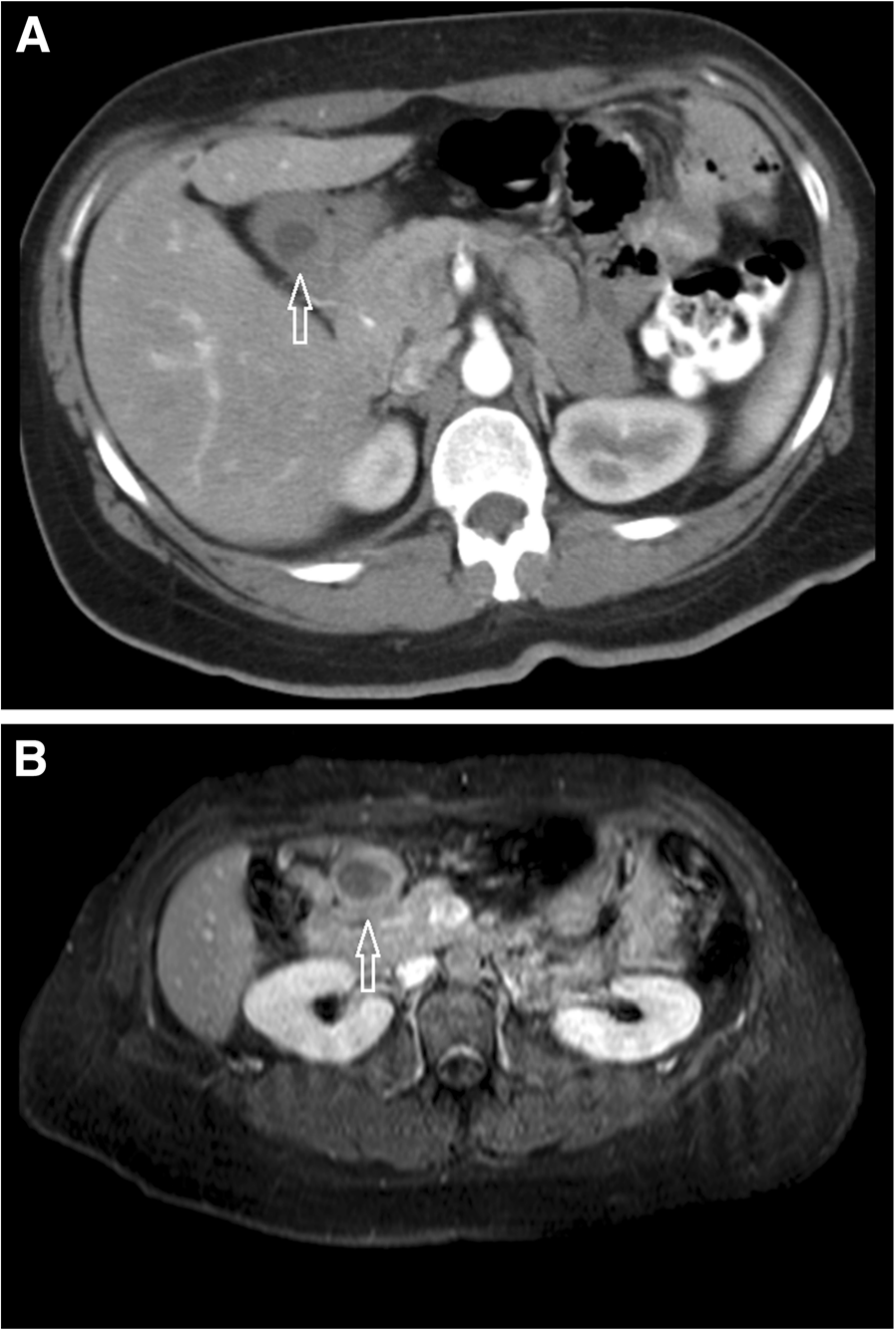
Abdominal MRI shortly after (A) and 5 years after gastric bypass (B) displaying the subserosal mass in the gastric antrum (arrows), the former previously unnoticed.
